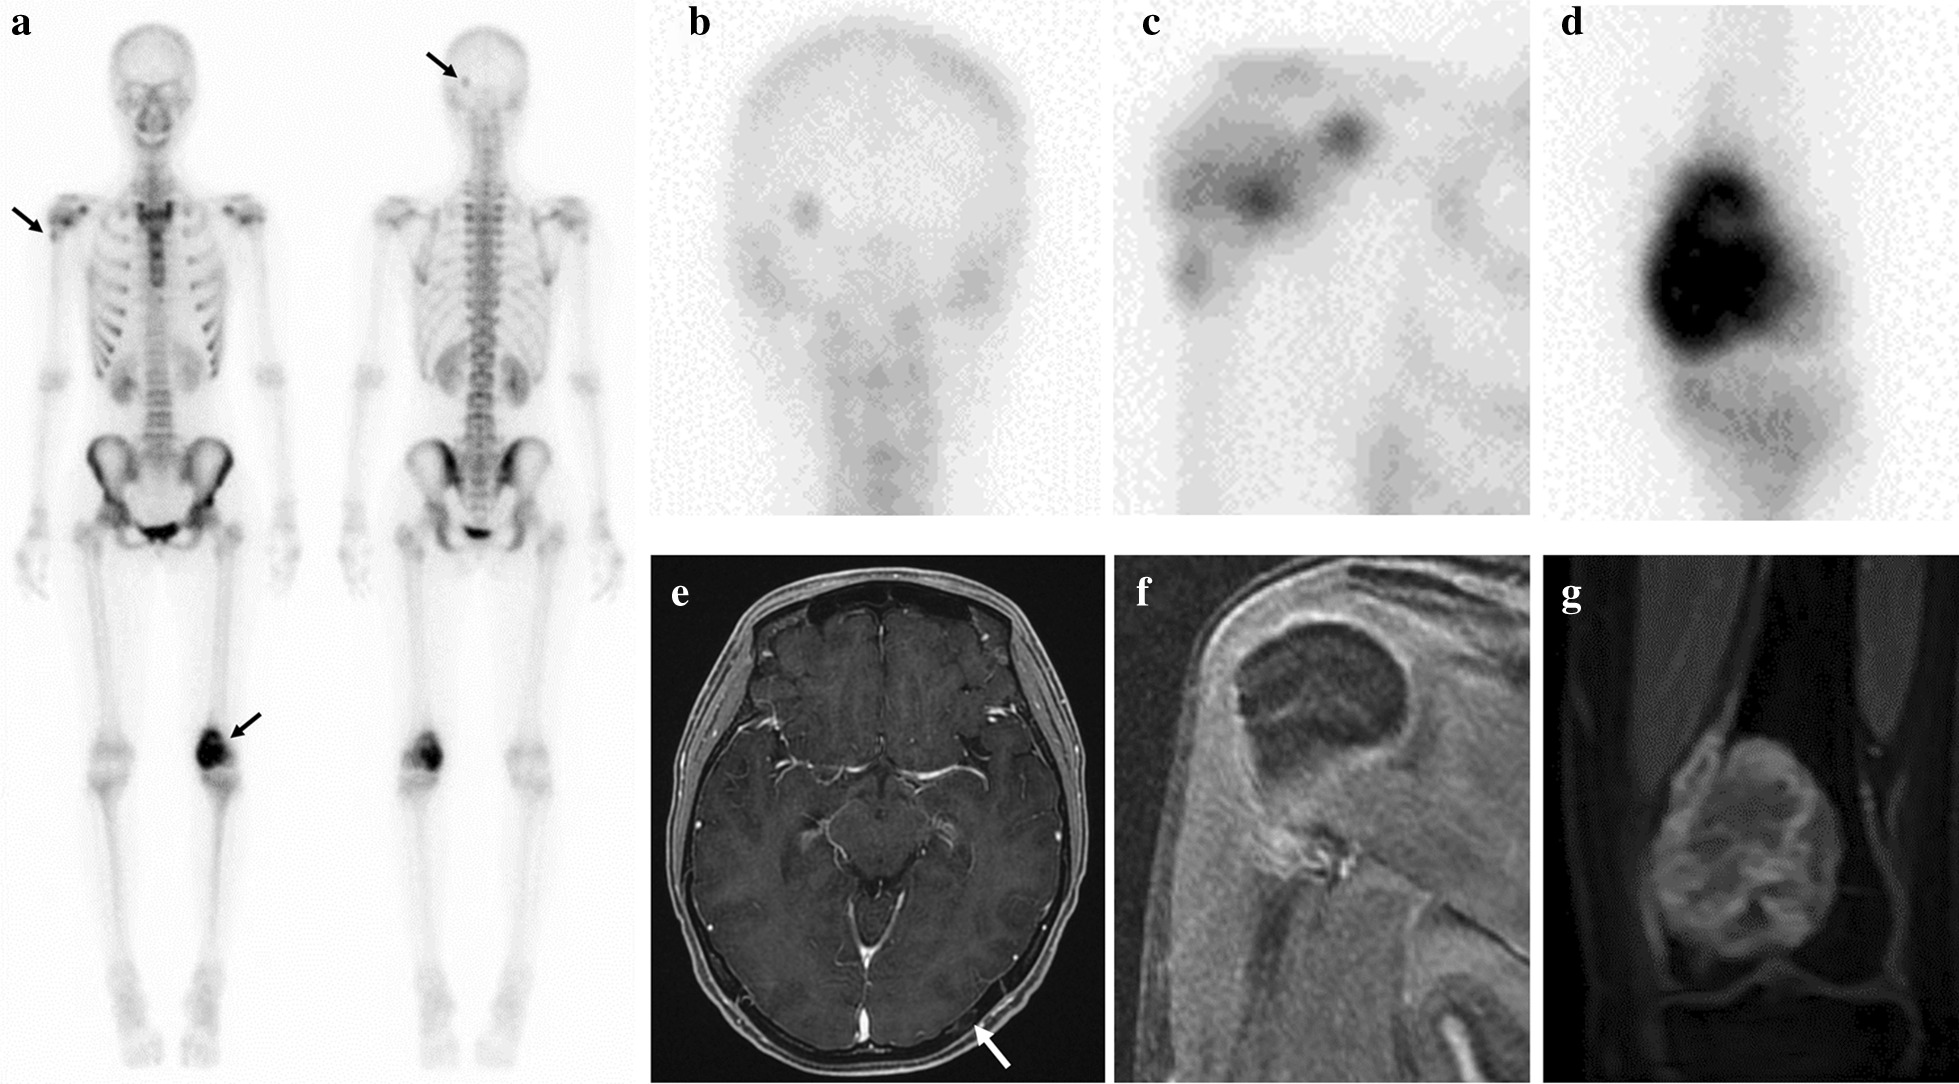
Systemic bone scan and MRI findings after five courses of neoadjuvant chemotherapy. a The uptake of all lesions on the bone scan was diminished. b Occipital bone lesion. c Right proximal humeral lesion. d Left distal femoral lesion. e The left occipital lesion had slightly shrunk in size on axial enhanced MRI (a white arrow shows the intracortical lesion.). f The enhancement was drastically decreased on coronal MRI of the right proximal humeral lesion. g The size and enhancement was decreased on coronal MRI of the distal femur
